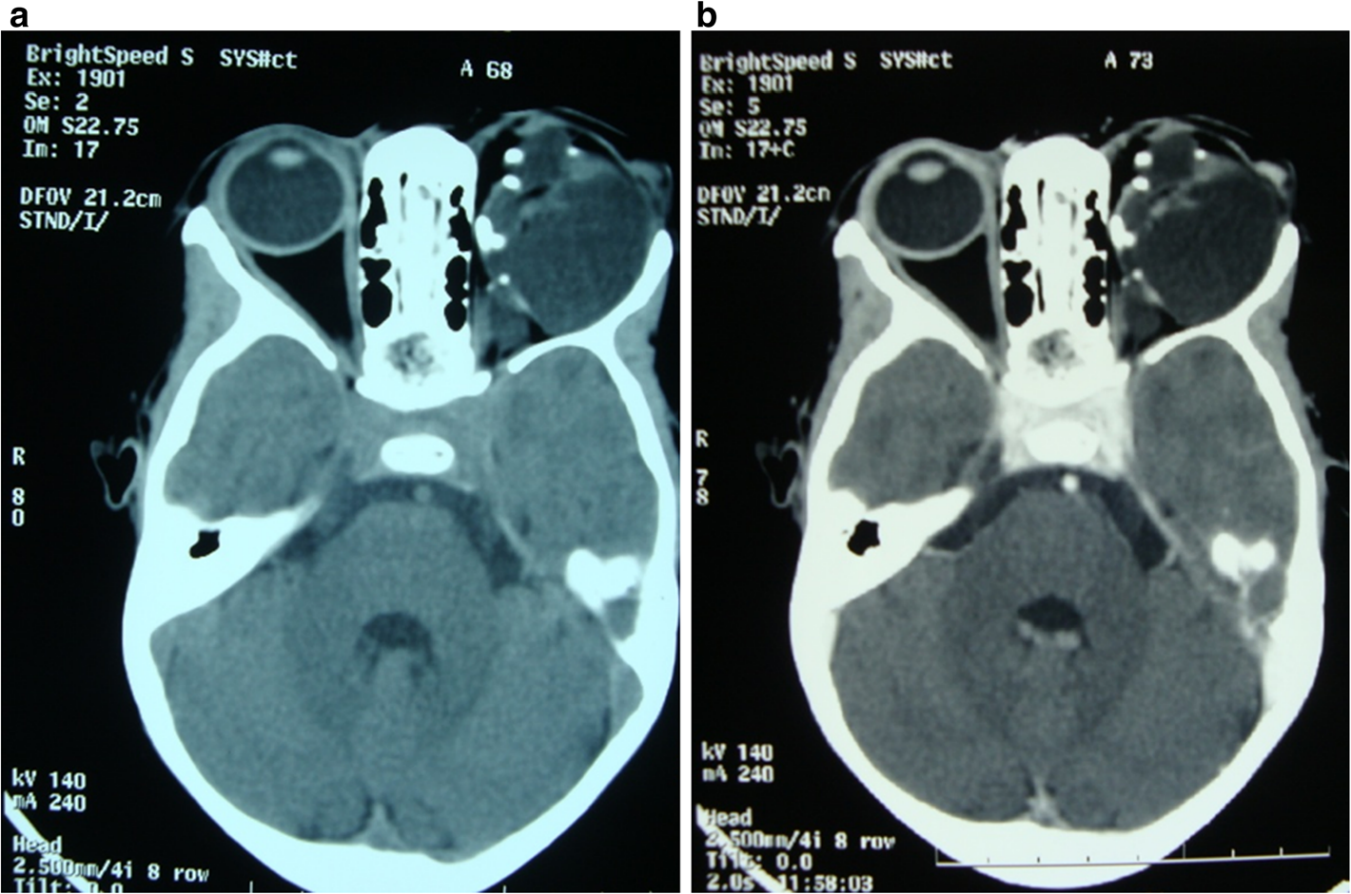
Computed tomographic scan. (a) This shows a huge, non-enhancing mixed density mass with areas of calcification. (b) The orbit is enlarged with thinned wall.
